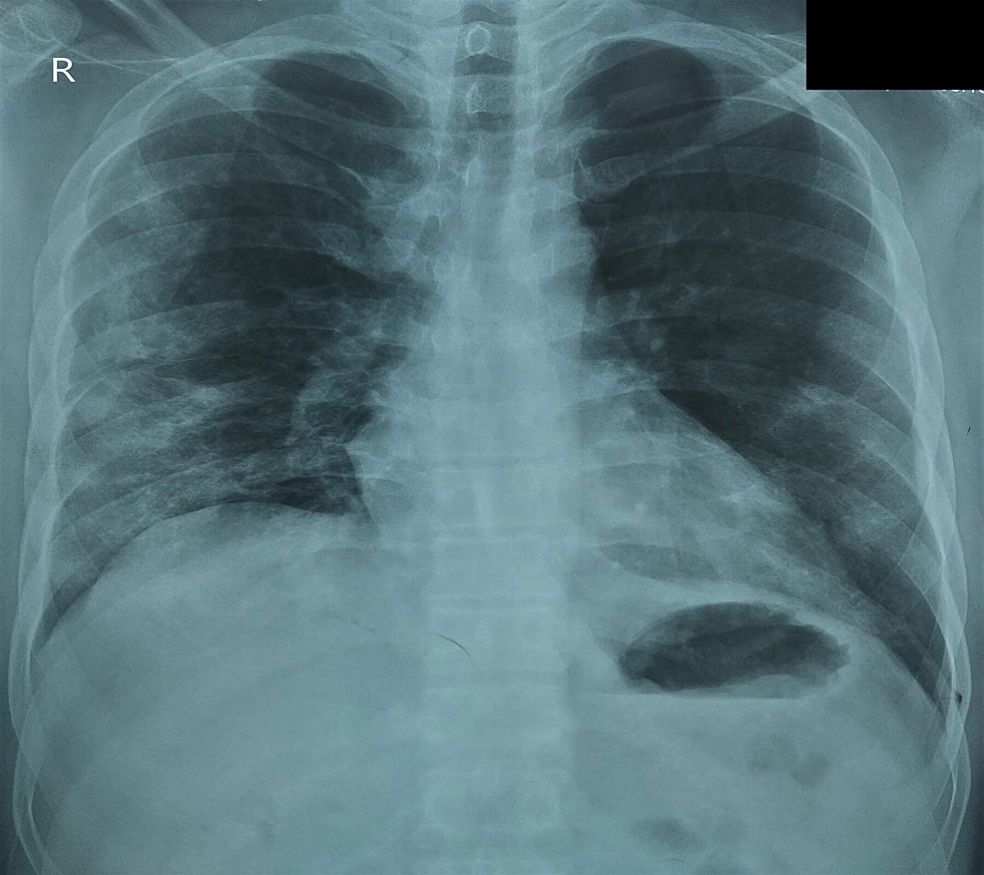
Chest x-ray posteroanterior view demonstrating bilateral (right more than left) peripheral patchy opacities.
